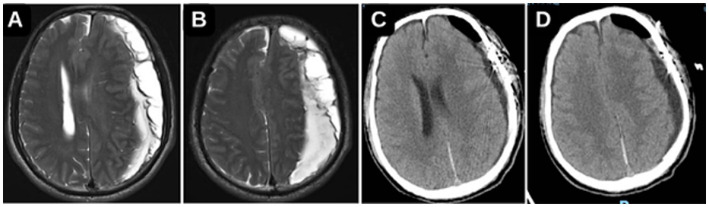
Radiological presentation and surgical outcome for case 3. (A,B) Preoperative imaging highlighting a large, highly loculated chronic subdural hematoma with a “honeycomb” appearance, demonstrating distinct multi-laminar stratification and separation. (C,D) The corresponding postoperative CT scan confirms 94% hematoma volume reduction and rapid brain re-expansion following the triple-modality intervention.
